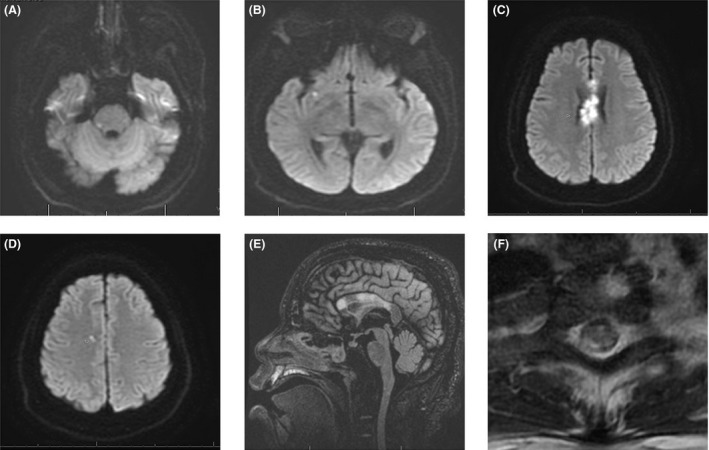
MRI without gadolinium. (A–D) Axial DWI MRI showing diffusion limited restricted diffusion involving right posterolateral aspect, right temporal region, corpus callosum, and right central semiovale. In addition, corpus callosum showing expansive lesions. There was also associated T2‐weighted and fluid‐attenuated inversion recovery (FLAIR) hyperintensities. (E) Sagittal FLAIR MRI showing hyperintense signals involving corpus callosum. (F) Axial T2‐weighted MRI cervical spine showing hyperintense signals involving posterior columns of cervical cord at C2 level.
